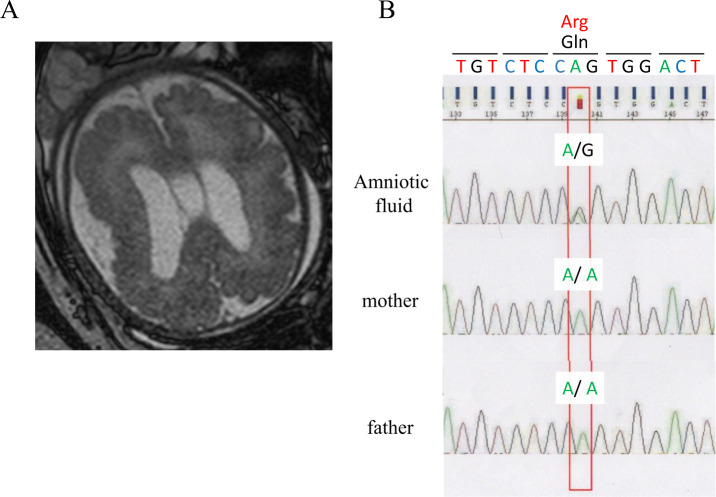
Fetal MRI findings in a patient with de novo heterozygous variant in the AKT3 gene. A Fetal magnetic resonance imaging (MRI) revealed that the fetus had asymmetrical megalencephaly with cortical dysplasia. B The chromatogram represents a *de novo* heterozygous c.233A > G (p.Gln78Arg) variant in the *AKT3* gene.
